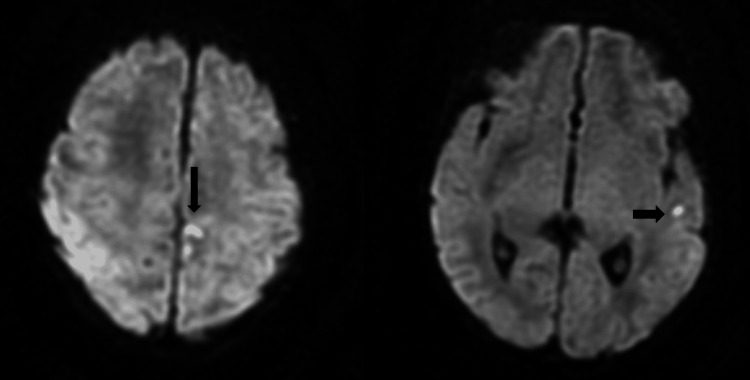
MRI brain diffusion-weighted imaging (DWI). MRI brain showed multiple areas of high DWI intensities, with associated low ADC signal intensities involving the bilateral parietal parasagittal region and right cortex (black arrows).
